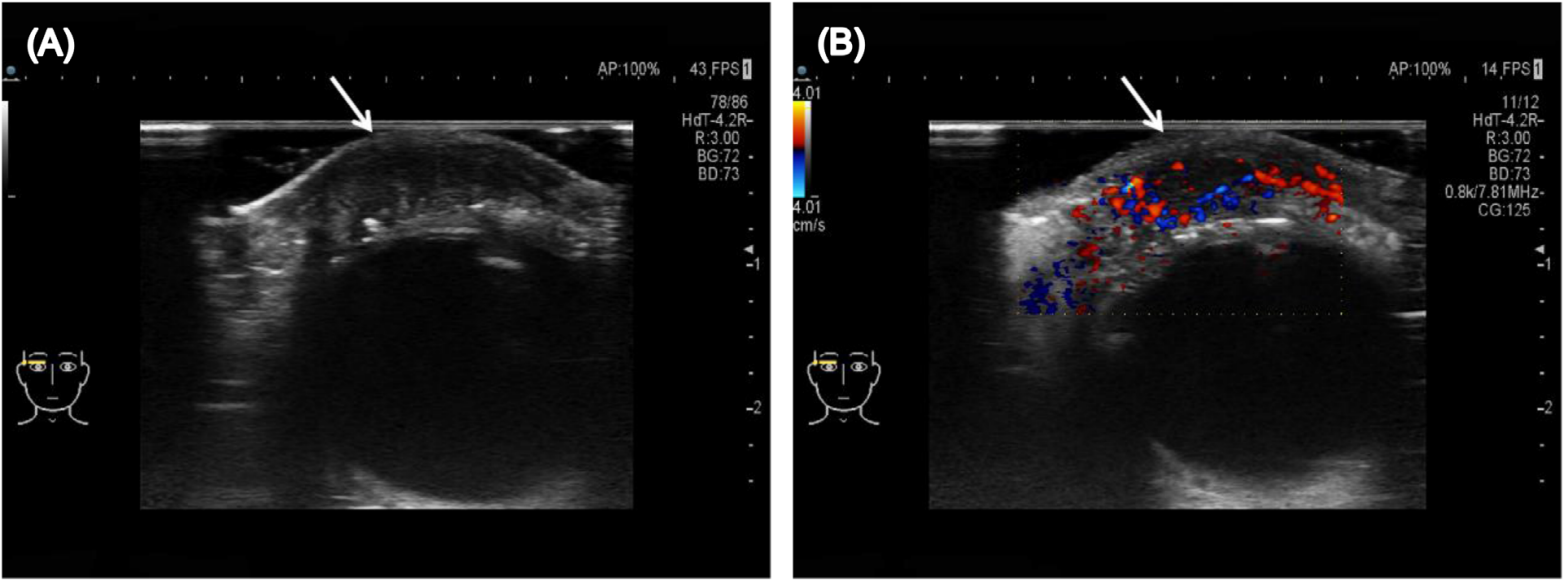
High‐frequency ultrasound of sebaceous carcinoma. (A) Grayscale ultrasound showed the solid mass located in the right eyelid was inhomogeneous, hypoechoic, ill‐defined, and irregular. (B) Color Doppler flow imaging showed abundant blood flow signals inside the mass.
